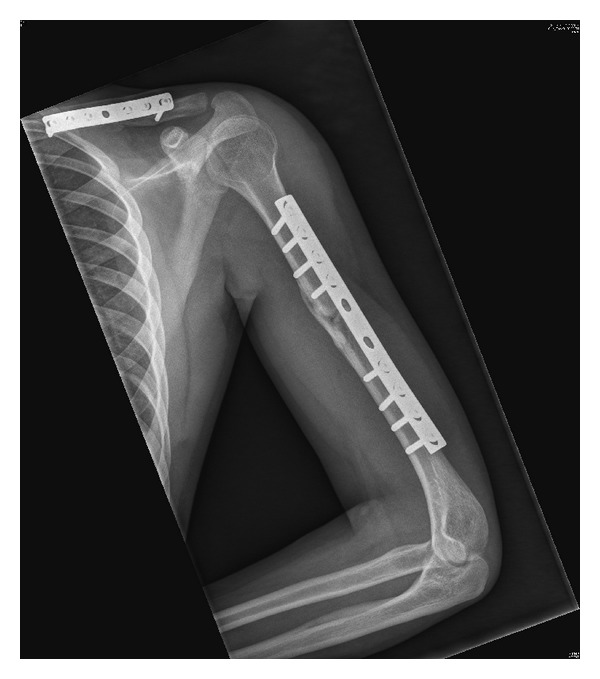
Follow-up radiograph showing union of the clavicle and humerus.
